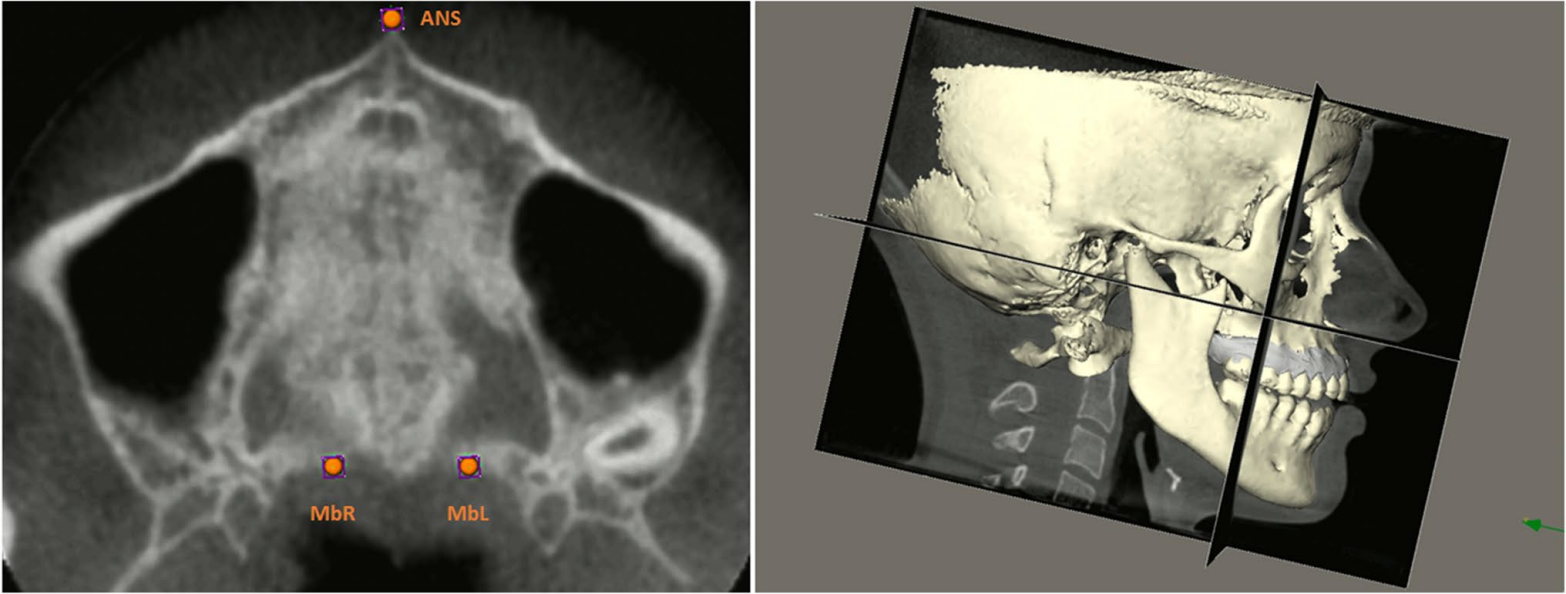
Orientation of the CBCT. (A: *left*) CBCT orientation of the horizontal plane defined by 3 landmarks: ANS-point, maxillary posterior border notch on the right (MbR), maxillary posterior border notch on the left (MbL). (B: *right*) CBCT orientation with 3D reference frame
